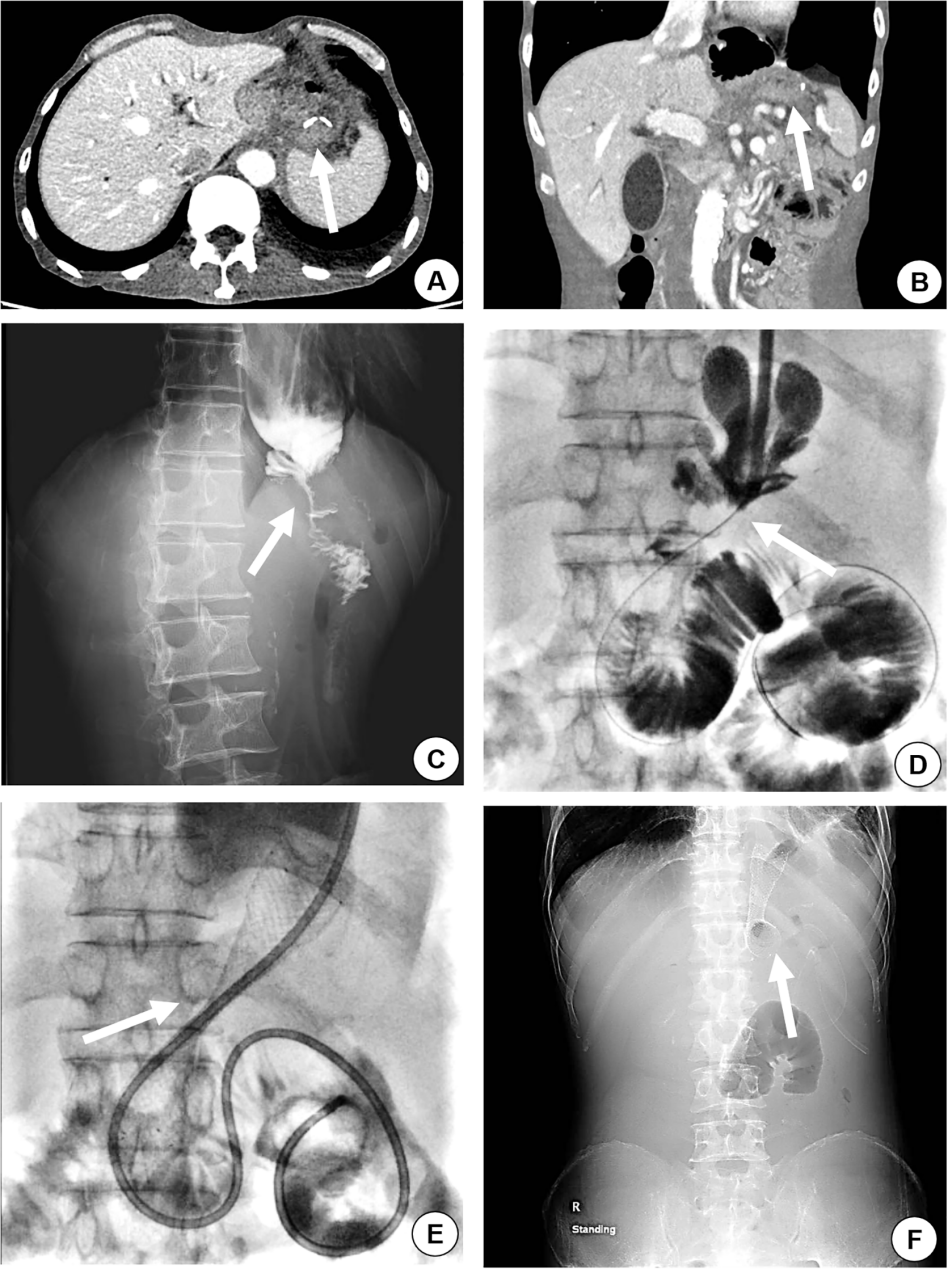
A 54-year-old male, with a history of total gastrectomy performed over 2 years prior, was admitted for recurrent nausea, vomiting, and failure to tolerate oral intake. Abdominal contrast-enhanced CT (axial and coronal planes) demonstrated anastomotic thickening and significant enhancement (arrow), radiologically suspicious for recurrent malignancy (A, B). Upper gastrointestinal contrast study revealed severe stenosis at the anastomotic site, with contrast agent passing through in a thin, thread-like stream (C, arrow). The iodinated contrast media was slowly injected to confirm the site of anastomosis stenosis (D, arrow), and the stent was placed in occluded segment of the site (E, arrow). A plain X-ray radiograph of the abdomen was taken to evaluate the site and dilation of the stent (F, arrow).
